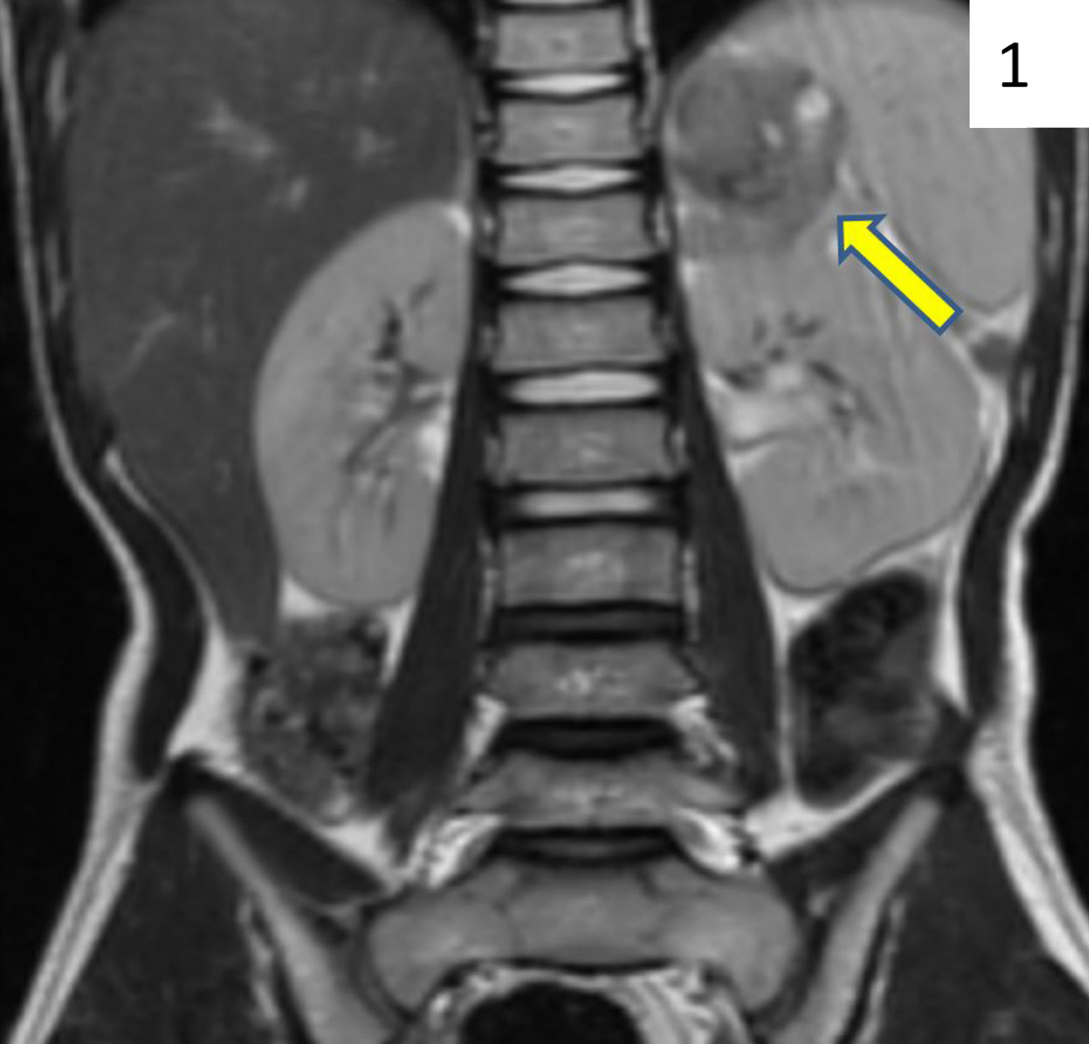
T2‐weighted MRI of abdomen, coronal view. The arrow indicates a 5‐cm lesion above the left kidney consistent with an adrenal mass.
